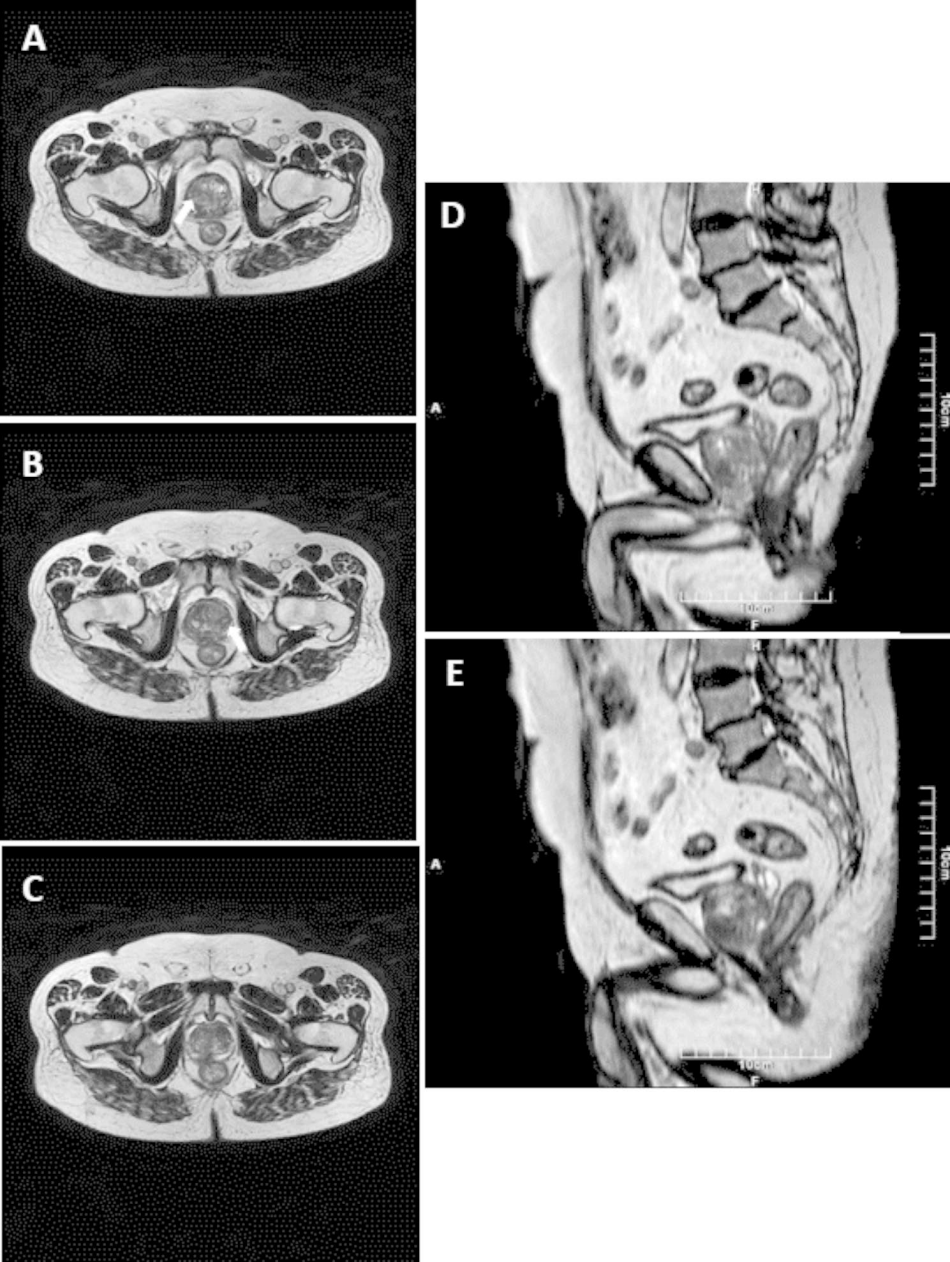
In MR imaging, which was also performed for planning, no obvious signal disruption was present due to the non-magnetic property of gold markers (same patient as Fig. 2). White arrows demonstrate the prostatic lesions
